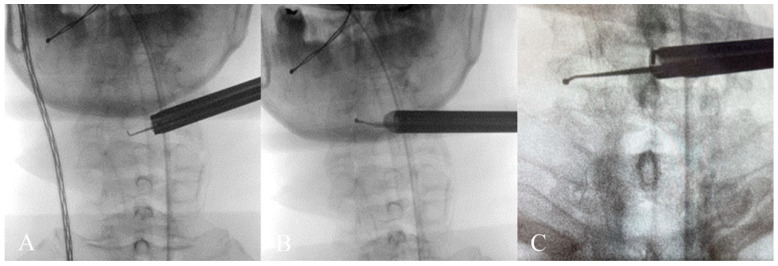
(A–C) depict intraoperative X-rays during contralateral laminar and foraminotomy decompression, demonstrating the extension of the spinal endoscopic instruments to the far edge of the contralateral foramen.
